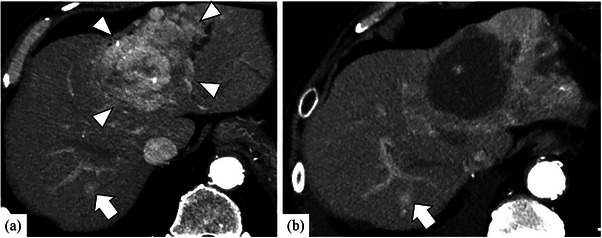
(a) Computed tomography during the arterial phase showed a tumor with 7 cm in diameter (white arrowheads) in liver segment 4. Computed tomography showed the high‐density area in liver segment 7 (white arrow). (b) Computed tomography was performed after the first transcatheter arterial chemoembolization, and the tumor in liver segment 4 could not be detected. Furthermore, it revealed that a tumor with a 1 cm diameter was detected in liver segment 7 as a variable hepatocellular carcinoma (white arrow).
